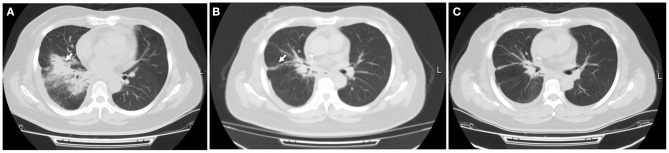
Radiation-induced pneumonitis. (A) After receiving three-dimensional thoracic radiotherapy, a CT scan showed new patchy opacity developed in the irradiated area of hilum of the right lung, which did not occur outside the irradiated area. (B) This patient initiated steroid treatment, pneumonitis was partially resolved within 1 month, with a significantly clinical improvement. (C) Pulmonary inflammation disappeared 2 months later. White arrow indicates inflammatory lesions.
